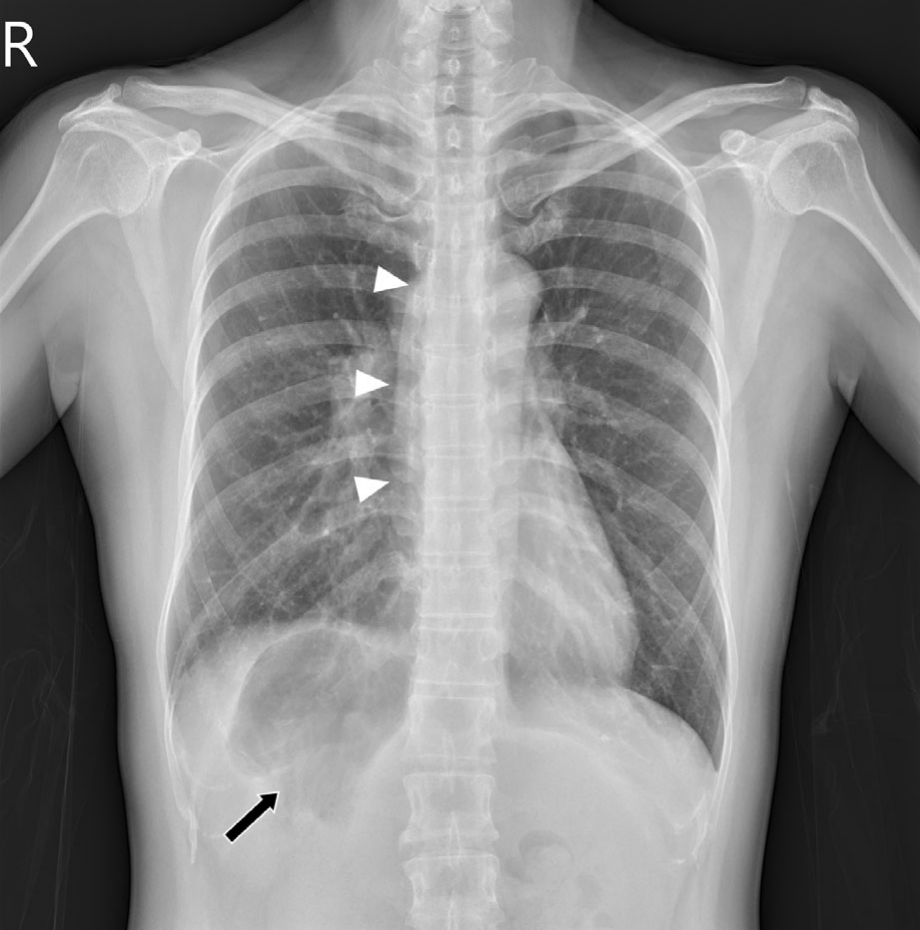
Poster‐anterior view of chest radiography showed abnormal gas collection below the right hemidiaphragm (arrow) and prominent azygous vein (arrowheads).
